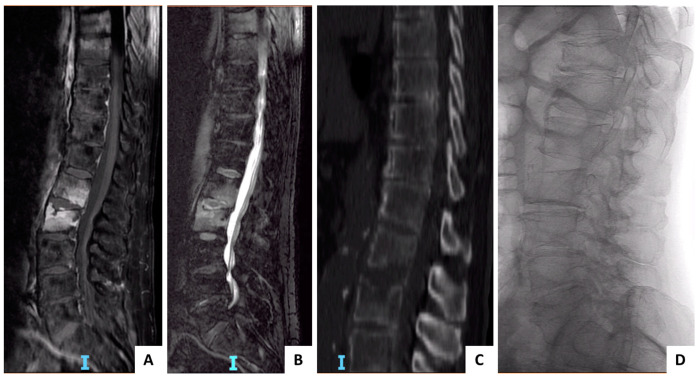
(A–D) Case example of L1–L2 pyogenic spondylodiscitis presenting without low back pain. An 86 years-old female patient with a history of chronic obstructive pulmonary disease (COPD), cigarette smoking, Alzheimer’s disease, and recent history of repeated bladder catheterization due to urinary retention. At presentation, the patient had been complaining of low-grade fever for the last 3 weeks and no low back pain. Blood tests show increased C-reactive protein (25 mg/dL) and white blood cell counts (12.4 × 10^3^/mm^3^). A chest X-ray followed by a chest CT scan were ordered at first but showed no signs of infection. Repeated blood tests showed raised gamma fraction in serum protidogram, but a whole body skeletal X-ray did not show any sign of osteolytic lesions. A whole spine MRI exam was ordered with a query diagnosis of multiple myeloma. MRI exam (panels A and B) showed the presence of an L1–L2 spondylodiscitis, which was further confirmed by CT scan (panel C). The patient underwent CT guided biopsy and *E. coli* was identified as the causative agent. IV antibiotic therapy with ceftriaxone was started for 3 weeks and followed by 3 more weeks of oral amoxicillin/clavulanic acid. Complete healing of the infection was achieved with no residual biomechanical instability on standing X-rays examination at the end of treatment (panel D).
